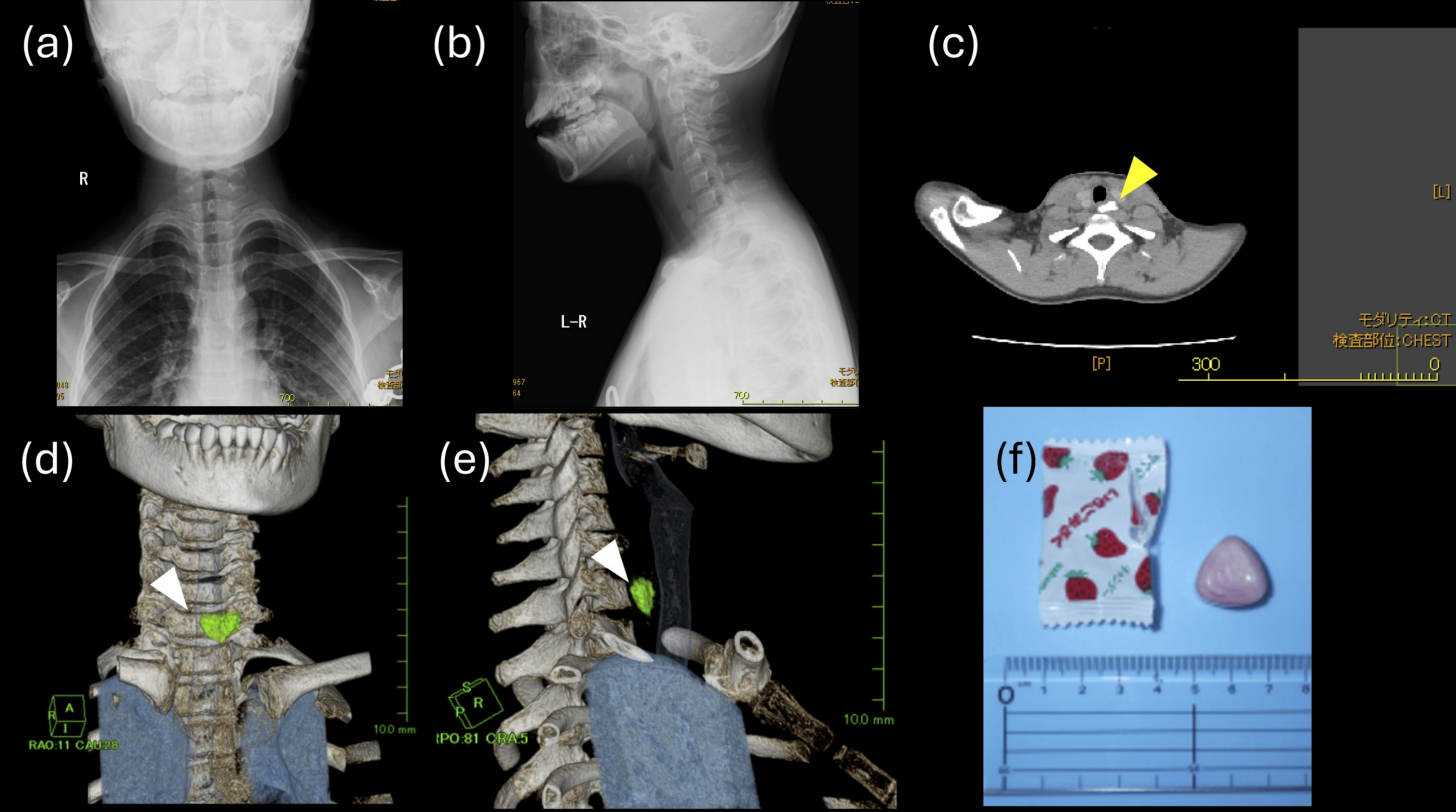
Imaging findings of the ingested foreign body (a) Frontal and (b) lateral radiographs showing no clear foreign body. (c) Axial CT showing the foreign body (yellow arrow) with a density similar to that of the vertebral bones. (d) 3D-CT reconstruction in frontal view and (e) lateral view showing the foreign body (green) lodged in the esophagus. The trachea is reconstructed in semi-transparent blue, confirming the absence of a foreign body in the airway. (f) The candy brought by the patient, matching the size of 18×15×8 mm and the shape of the foreign body visualized on the 3D-CT. CT: computed tomography; 3D-CT: three-dimensional computed tomography
